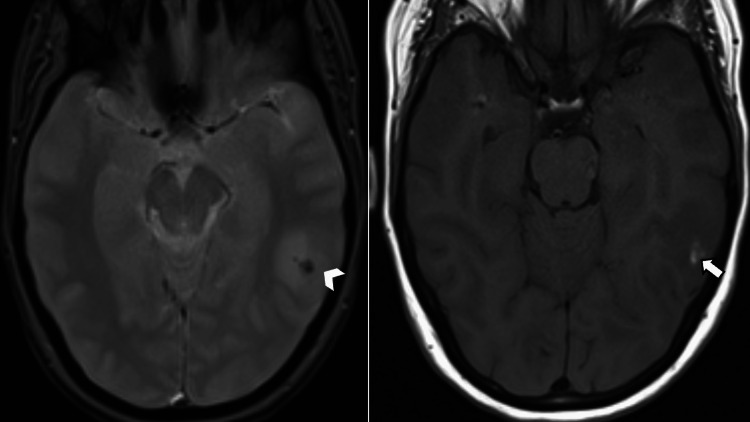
Gradient echo T2-weighted and T1-weighted sequences showing a left parietal hemorrhagic spot (arrowhead), which appears as a hyperintense signal on the T1 sequence (arrow).
